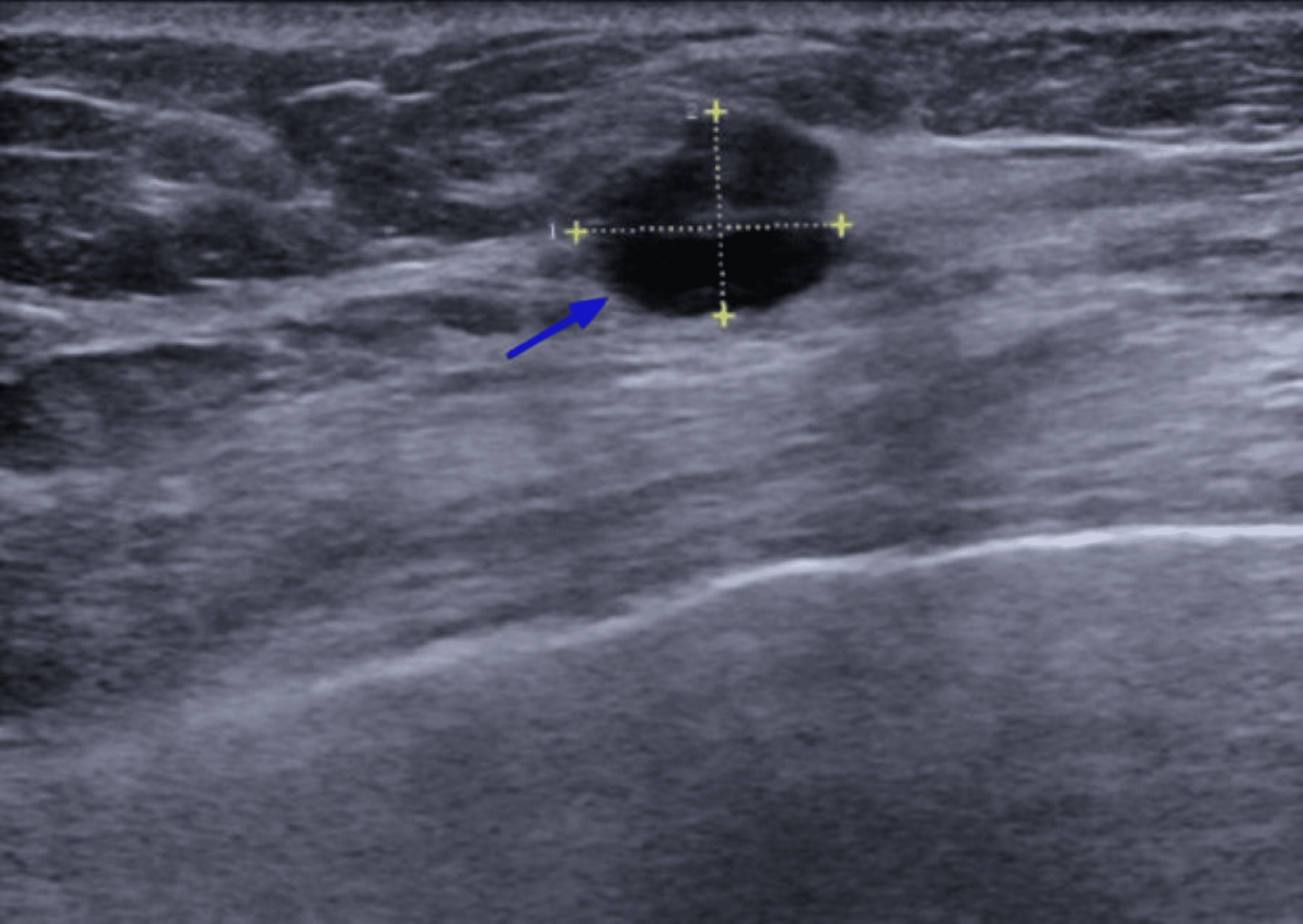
Ultrasound of the right breast showed a 1.2 x 0.9 x 1.4 cm suspicious age-related mass (blue arrow) at 10-11 o'clock position, 5 cm from the nipple. Both axillae are imaged and are normal.
